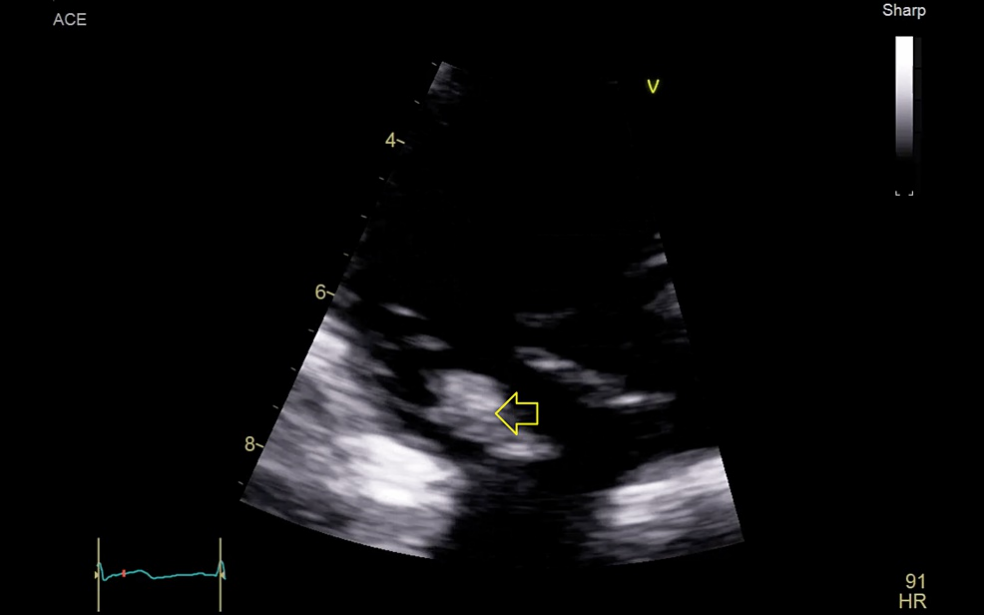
An image of the tricuspid valve of the first transthoracic echocardiogram showing a large mass (arrow) attached to a tricuspid valve leaflet.
